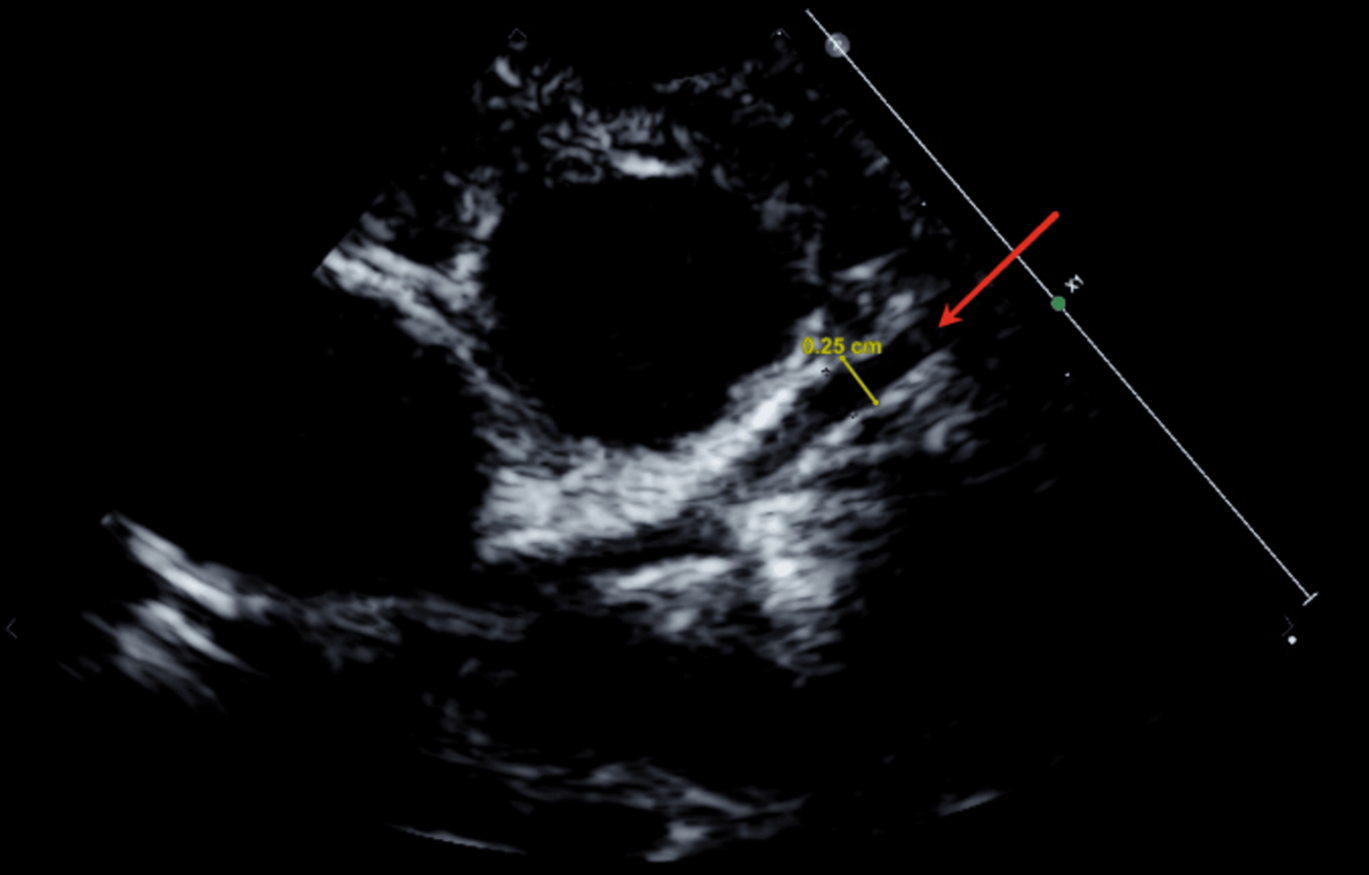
Echocardiogram from the parasternal short-axis view showing a dilated right coronary artery Red arrow pointing at a dilated right coronary artery measuring 0.25 cm
